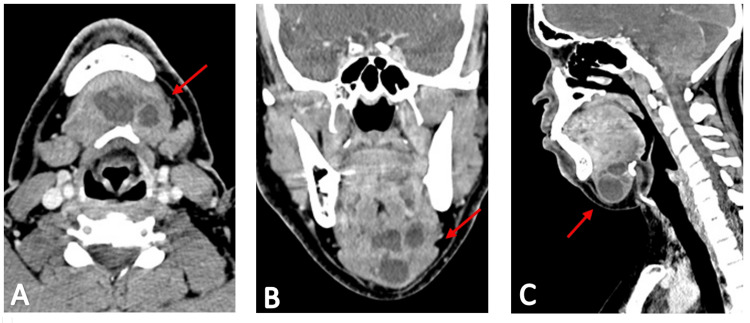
Axial (A), coronal (B), and sagital (C) views of CT scan showing a cystic mass Head and neck CT scan showing a large cystic mass (arrows), compatible with thyroglossal canal cyst. CT: Computed tomography
